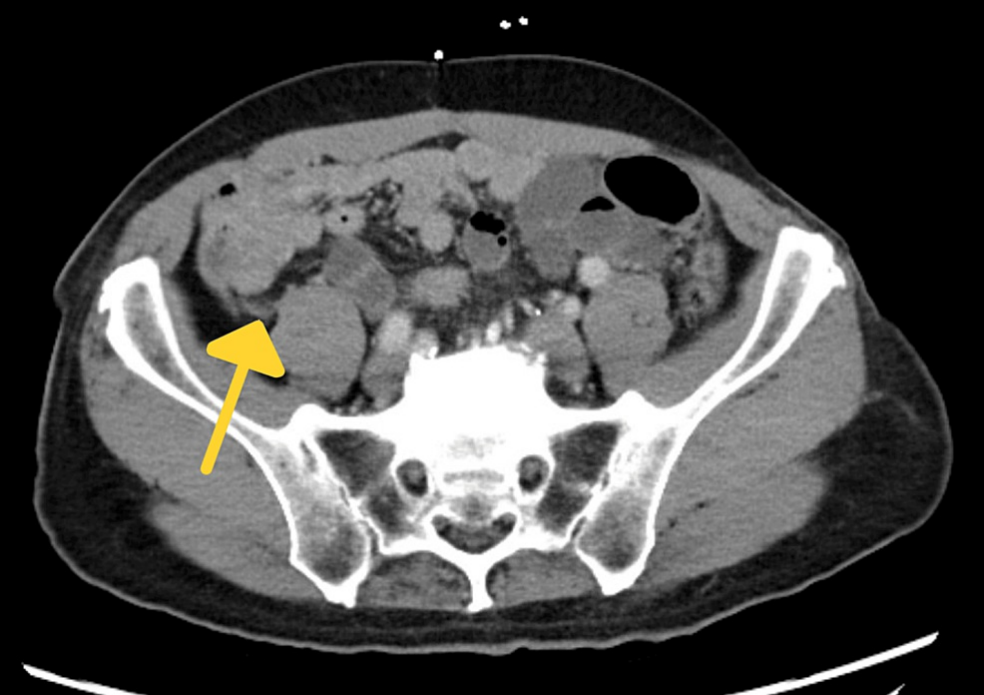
CT with arrow indicating appendix
